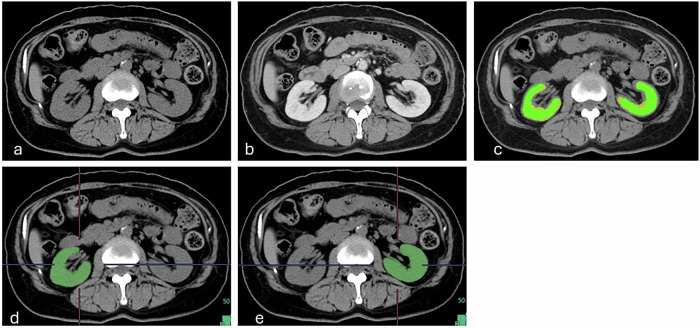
CT of an IgG4-RKD–negative case (female, 53 years). a Unenhanced CT of the kidney; b CE-CT of the kidney; c ROIs for texture extraction by MH (yellow) and JI (green); d, e segmentations (green) of the right and left kidneys by MH. CE-CT, Contrast-enhanced computed tomography; CT, Computed tomography; I gG4-RKD, Immunoglobulin G4-related kidney disease; ROI, Region of interest
